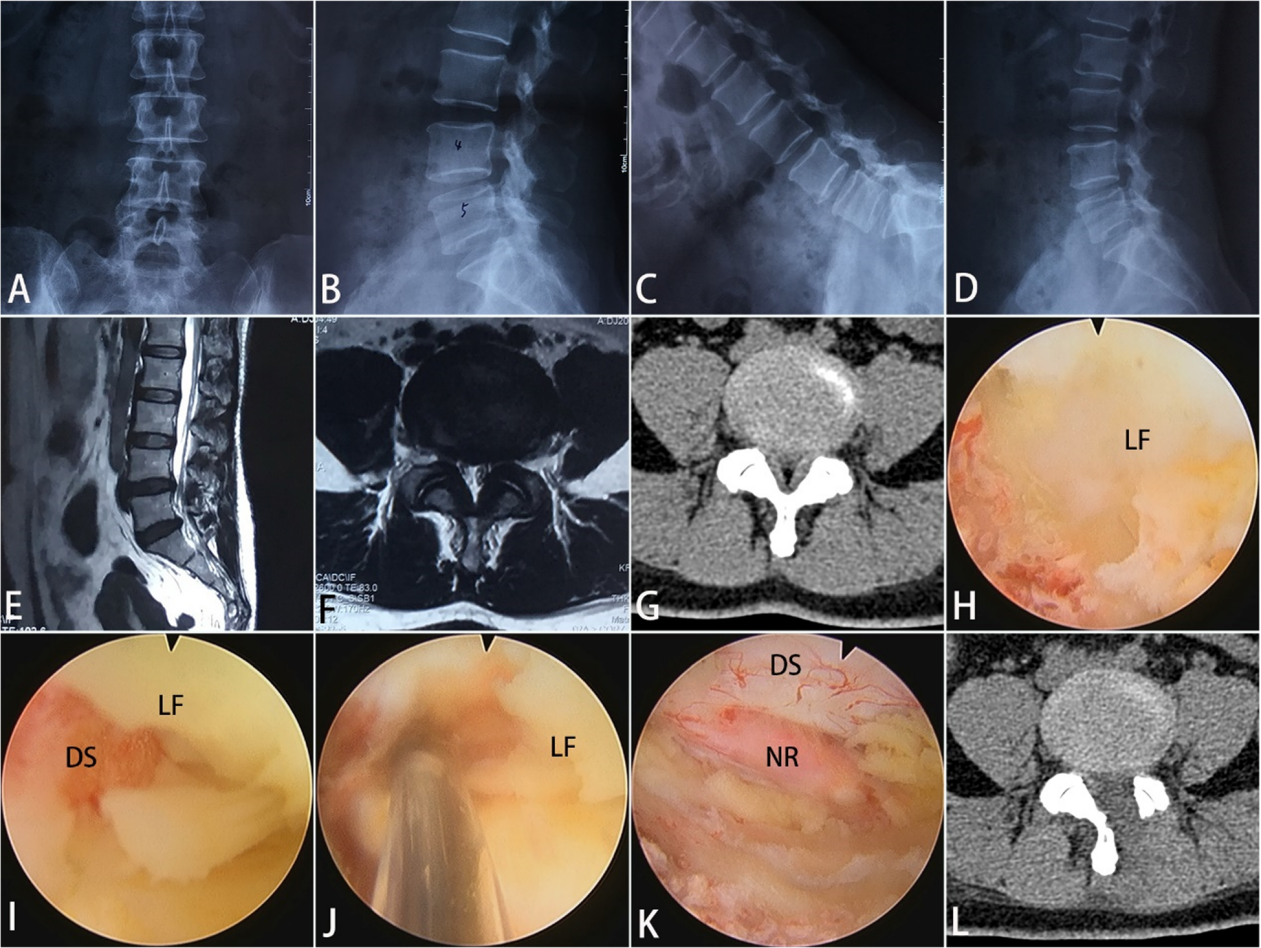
Lumbar endoscopic unilateral laminotomy bilateral decompression performed on a 51-year-old female patient diagnosed with L4-L5 lumbar spinal stenosis without degenerative spondylolisthesis or deformity. a and b preoperative anteroposterior and lateral plain radiographs; c and d preoperative flexion and extension radiographs; e and f preoperative magnetic resonance imaging scans; g preoperative computed tomography (CT) scans; h undercutting of the ipsilateral and contralateral cranial lamina was performed; i the ipsilateral and contralateral ligamentum flavum (LF) was identified and removed piecemeal with endoscopic punches and forceps; j the bipolar radiofrequency electrocoagulator was used for hemostasis; k adequate decompression of the ipsilateral and contralateral traversing nerve root (NR) and the dural sac (DS); l CT scans one week after the surgery
